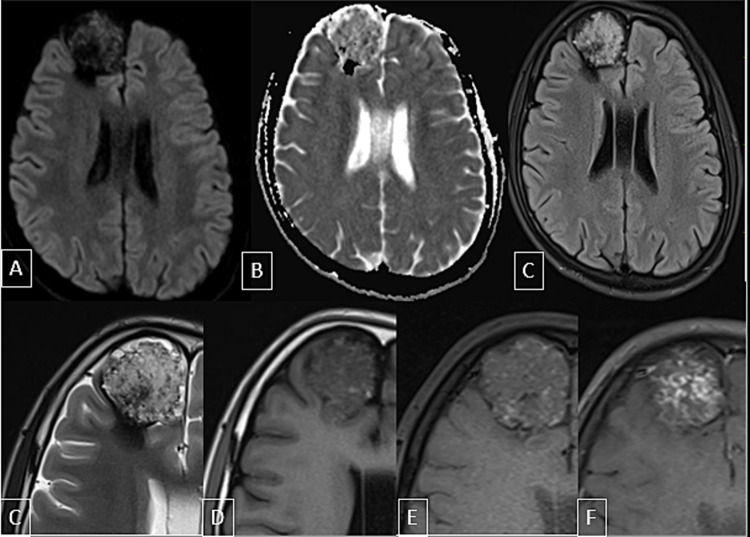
MRI brain scan. The scan showed a large well-defined cortex-based lesion in the anterior right frontal lobe in the right parasagittal plane showing no diffusion restriction (A-B). This lesion is appearing heterogeneously hyperintense on FLAIR (C) and T2-weighted images(D); iso to hypointense  on T1-weighted images (E). Multiple small T2 hypointense and T1 hyperintense foci are seen within the lesion (D, E). This lesion shows heterogeneous post-contrast enhancement on post-contrast T1FS images (F). FLAIR: fluid-attenuated inversion recovery; T1FS: T1-weighted fat-suppressed
